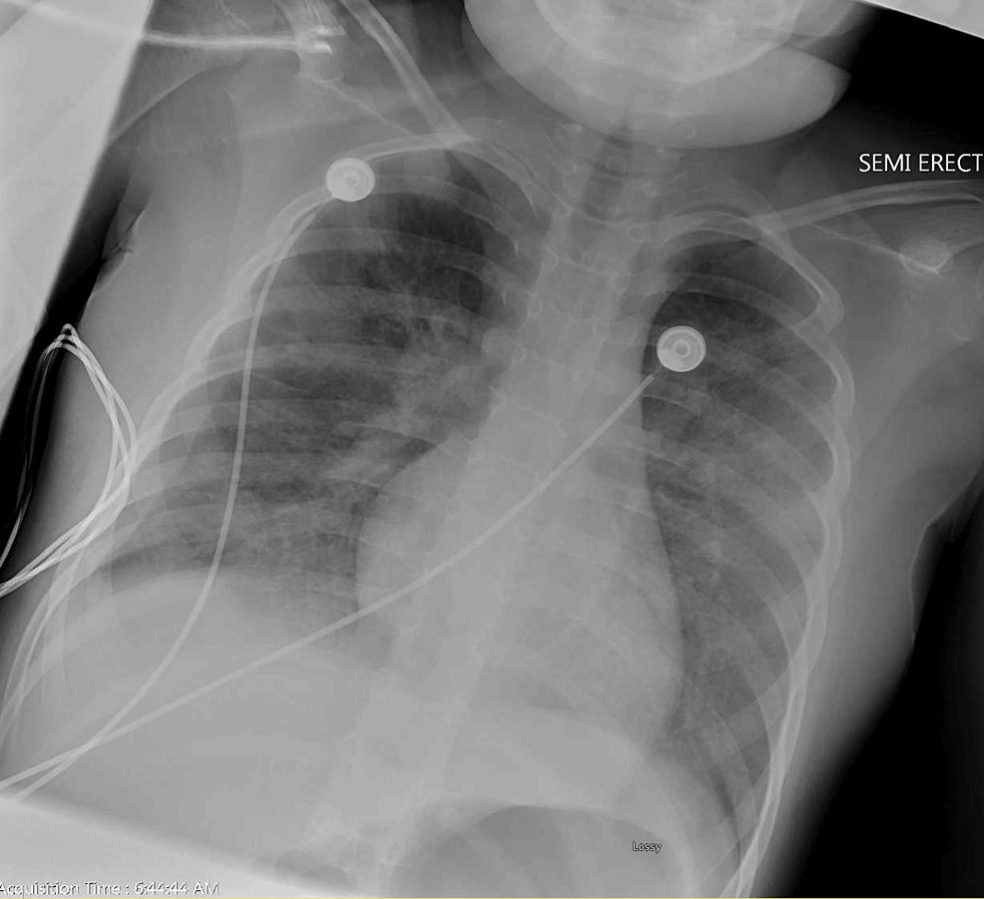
The patient's chest X-ray showed signs concerning for pulmonary edema.
